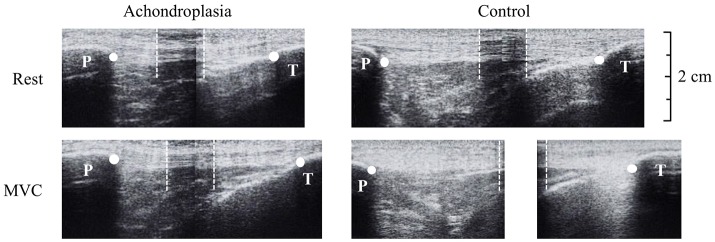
Ultrasound images during tendon elongation measures of the Achondroplasic (left images) and control (right images) patella tendon from rest (top) to maximal voluntary contraction (bottom). White dots represent the measurement of tendon length from patella tendon origin (patella, P) and insertion (tibia, T). For the longer tendons that exceed the ultrasound probe's field of view, an echo-absorptive marker was used as a reference point to help stitch images together (Onambélé et al., 2007). The boundaries of the marker are identified by the white dotted lines.
